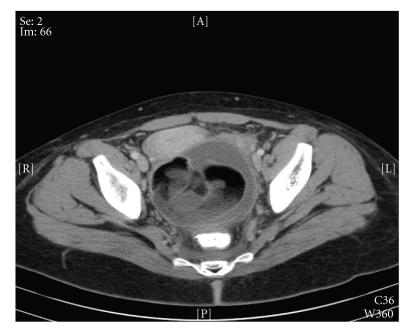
Computed tomography (CT) scan of the pelvic area showing a 10 cm × 9.5 cm mass in the cul-de-sac containing heterogenous echogenicities consisting of fat, fluid, soft tissue, and calcifications.
